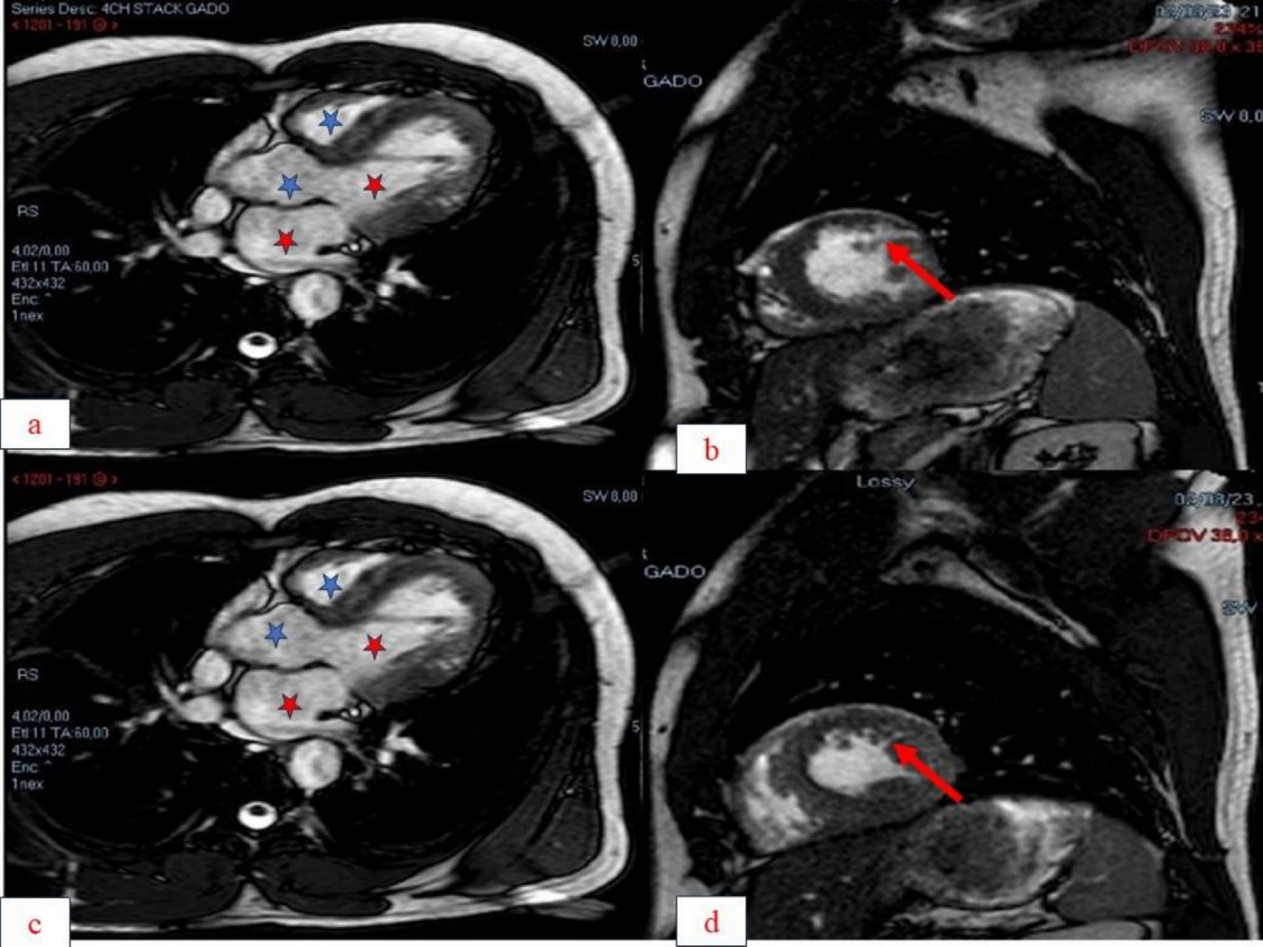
Cardiac MRI showing hypertrabeculation of the lateral, medial, and apical wall in favor of cardiomyopathy due to non‐compaction of the left ventricle. (a) and (c) Long‐axis incident view showing left cavities (red star) and right cavities (blue star). (b) and (d) Short‐axis view showing a vascularised recess (red arrow).
